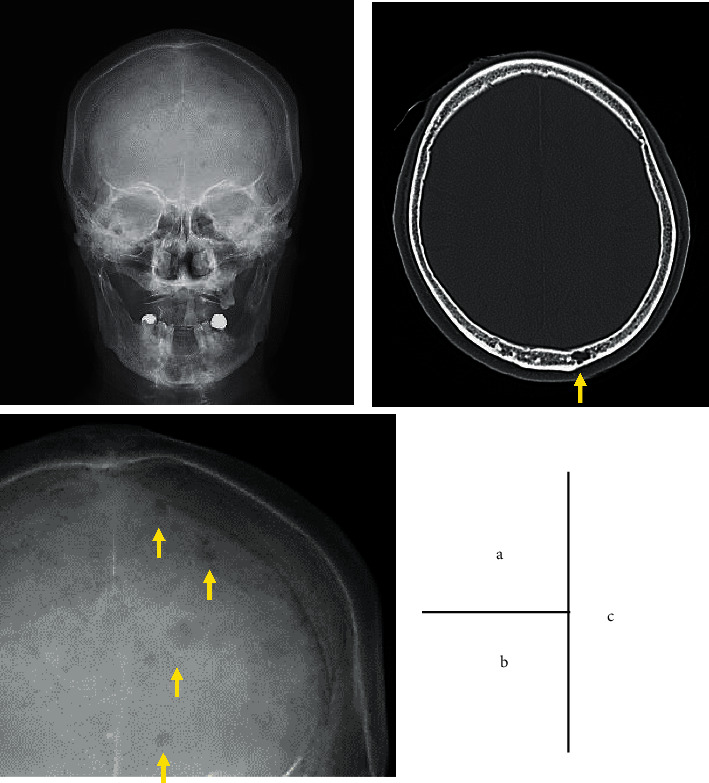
Osteolytic lesions of the skull. Head skeletal X-rays (a and b) and computed tomography (CT) (c) showed multiple, lytic, and punched-out lesions in the skull (arrows).
